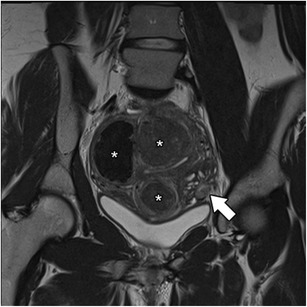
Subserosal endometriosis: Coronal T2-weighted image; subserosal ill-defined mass of low signal intensity, with high-intensity myometrial foci, in the left uterine wall from pelvic deep endometriosis. Leiomyomas are also seen (asterisks)
